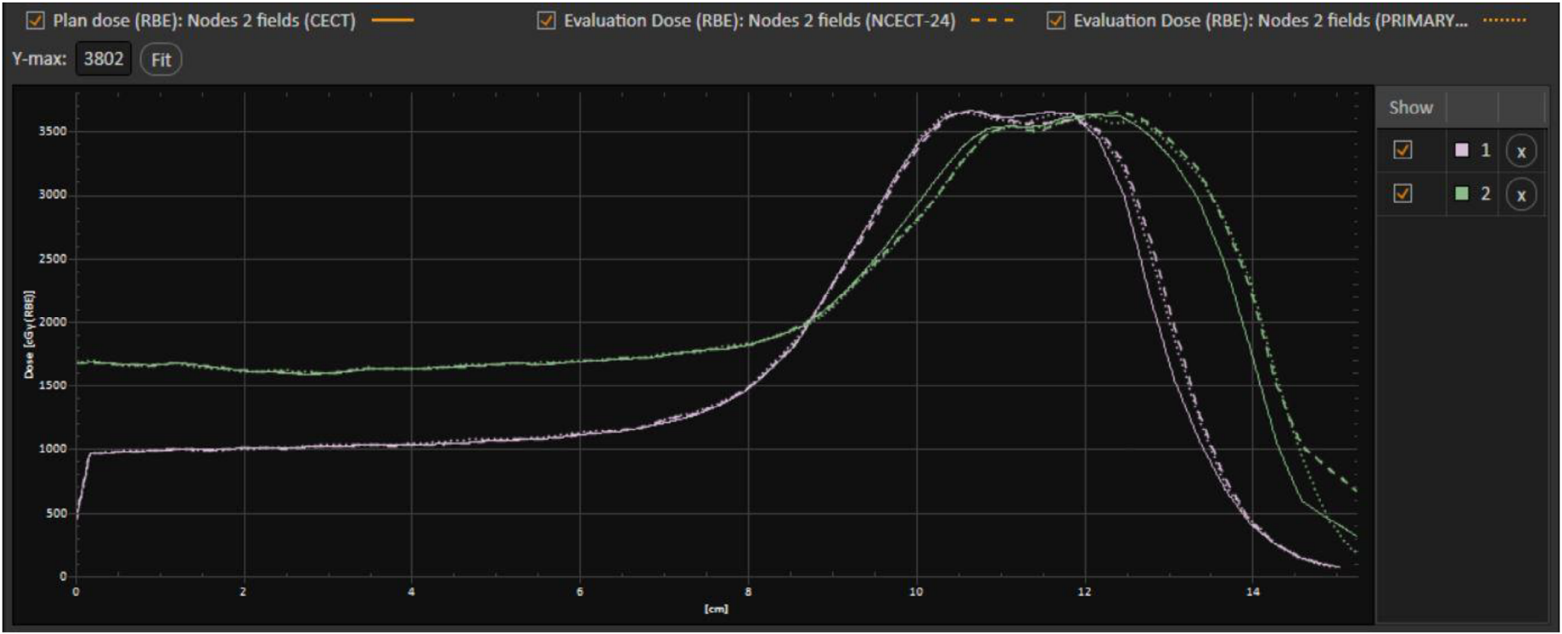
A comparison of the line dose along beam centers of two fields for plans generated using the CECT (solid line), the generated NCECT (long dash line), and the true NCECT, that is, the planning CT without contrast (short dash line).
